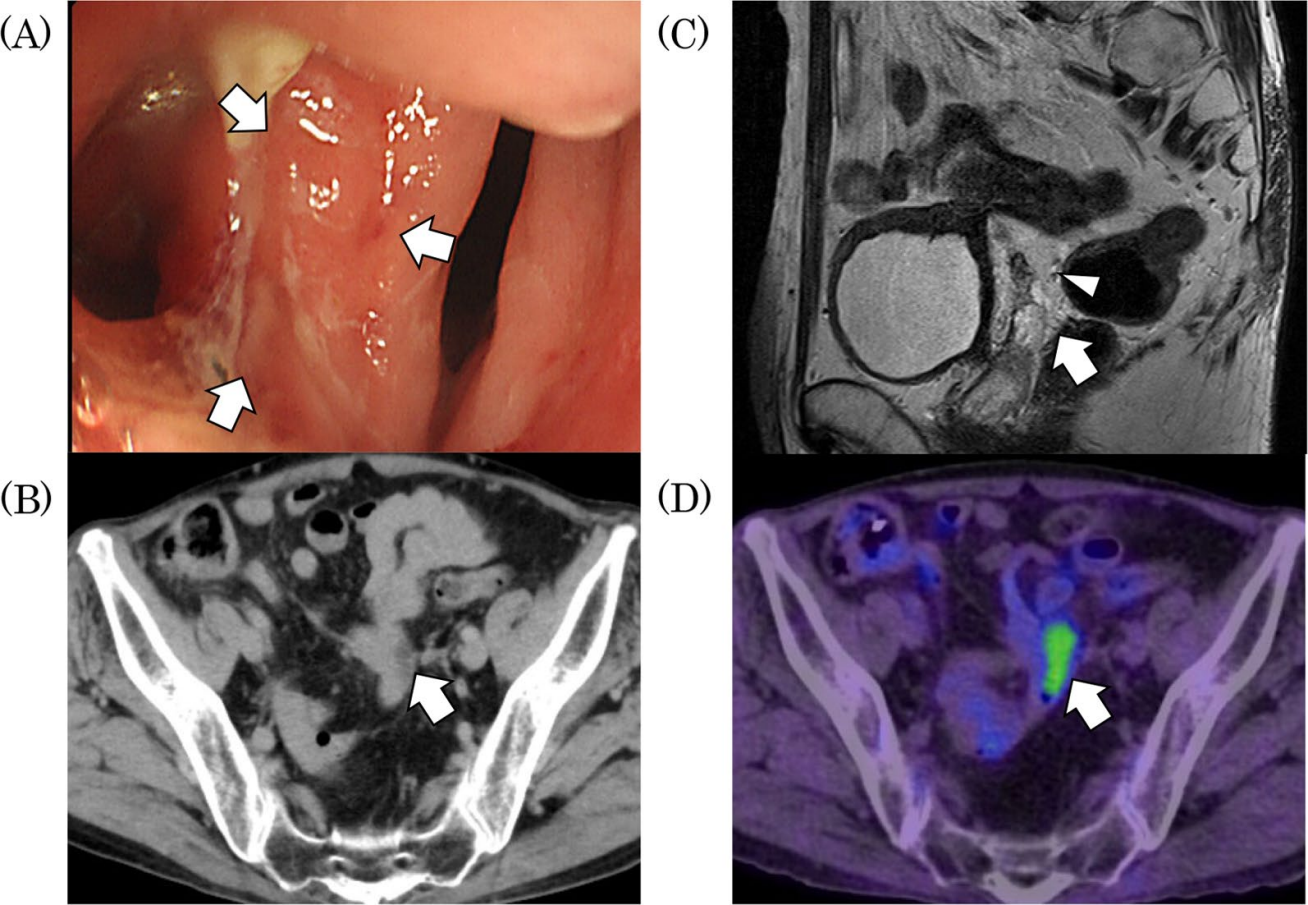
Imaging after treatment with pembrolizumab in Case 2. A Endoscopic imaging showing a sigmoid colon tumor turned fistula. B CT scan, after ipilimumab and nivolumab treatment, showing the tumor’s notable shrinking. C Magnetic resonance imaging (MRI) showing the fistula (arrowhead) between the sigmoid colon cancer and ileum. D PET–CT after ipilimumab and nivolumab treatment showing a low signal on the borderline between the sigmoid colon cancer and the bladder. Arrows identify the tumor
